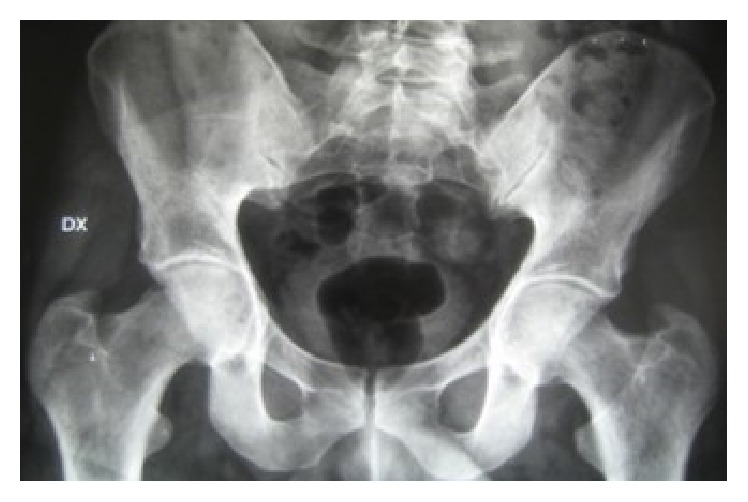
AP X-ray of the pelvis detects acetabular protrusion in a 36-year-old patient affected by Marfan syndrome. The patient complained of hip pain and clinical examination showed a restricted range of motion of the hips.
